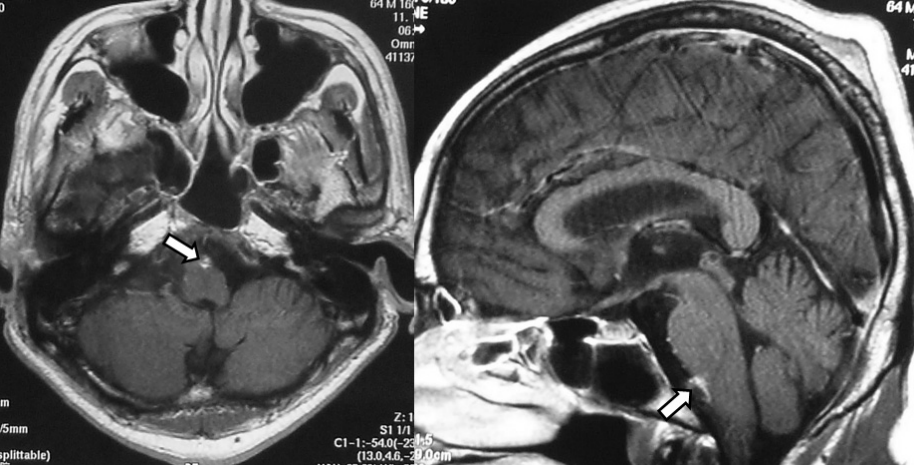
MRI of the brain was performed again two months after surgery. This time it revealed disseminated lesions in the medulla oblongata.
